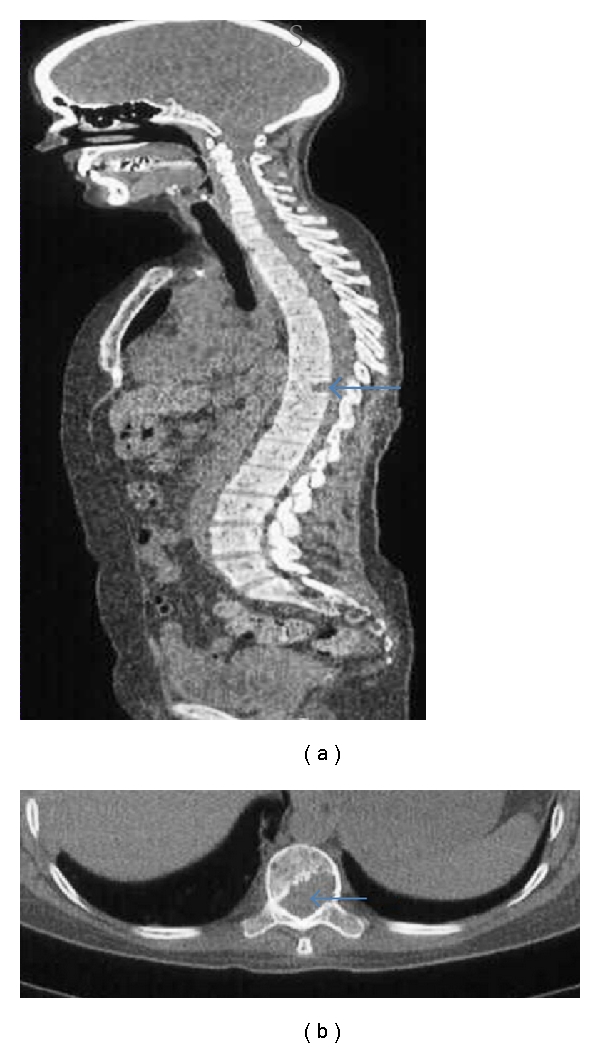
Low dose sagittal whole-body CT (a): note lytic lesion posterior aspect of T10 vertebral body (arrow). Background of extensive osseous permeation from myeloma. Axial CT thorax in the same patient at the level of T10 (b) identifying lytic infiltration of vertebral body (arrow).
